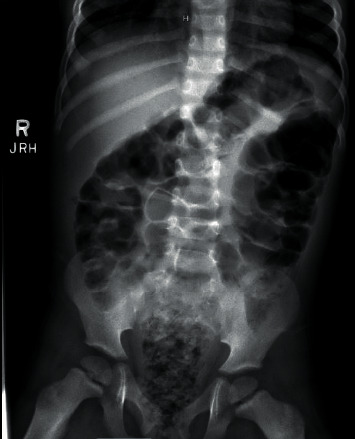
Abdominal X-ray, supine view. Following 2 days on stool softener and representation to the ED, there is mild gaseous distention of the visualized large colon with a moderate degree of fecal material within the rectum and rectosigmoid colon, not subsequently moved by mineral oil enema.
